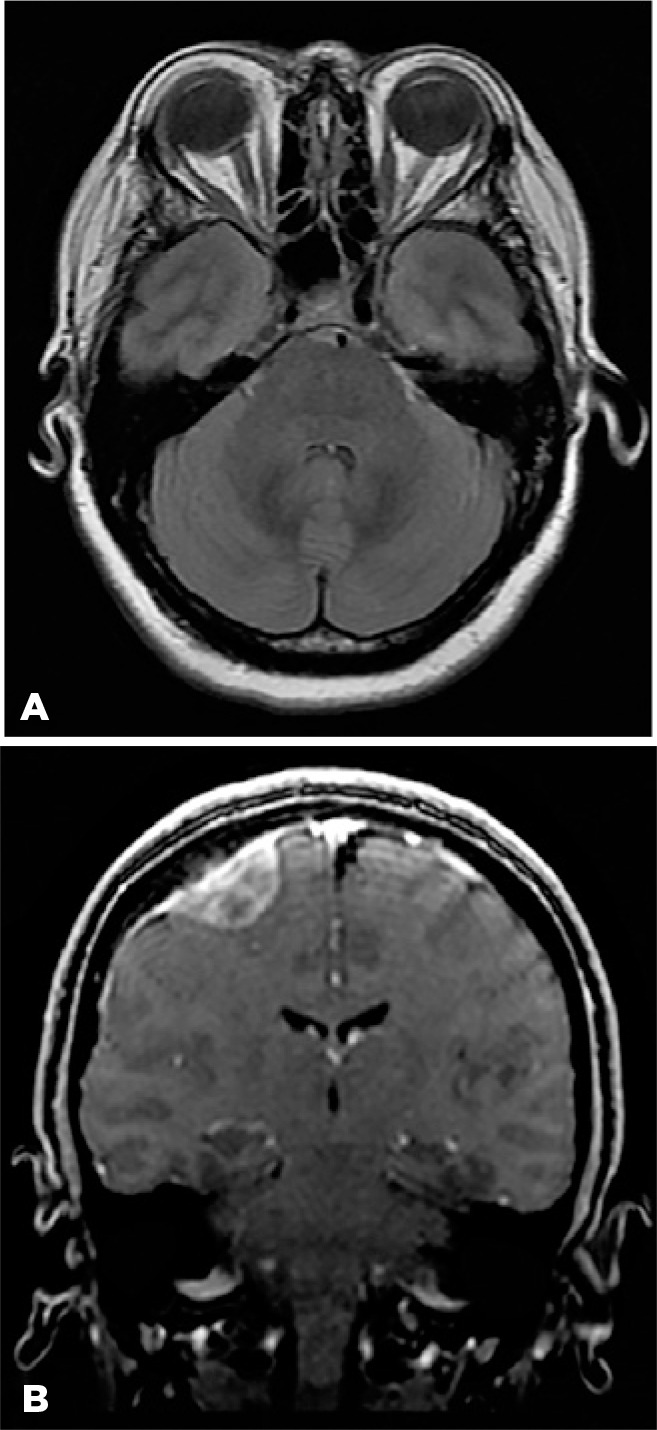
Cranial magnetic resonance imaging reveals flattening of the posterior
